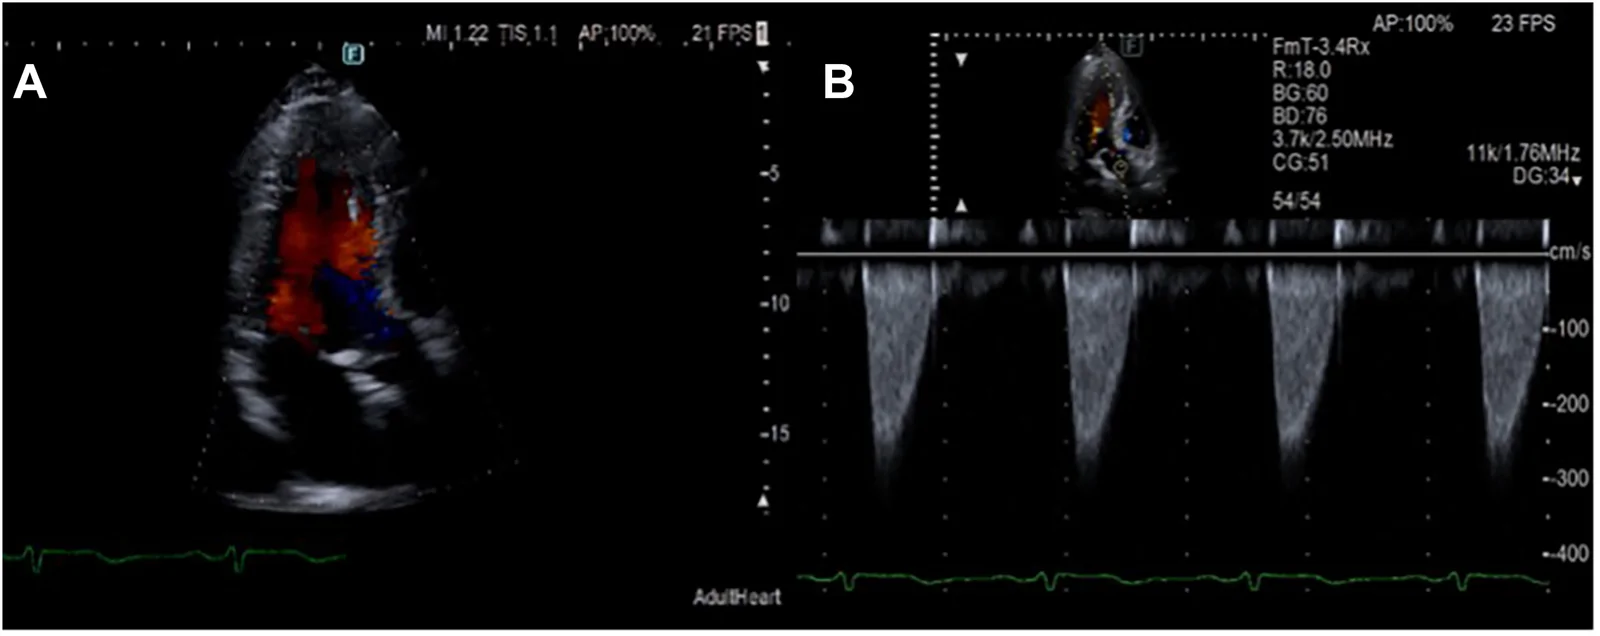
Transthoracic echocardiography at 6.5 years postoperatively. (A) Apical long-axis view showing trace aortic regurgitation. (B) The mean pressure gradient was 15 mm Hg, indicating no evidence of stenosis.
